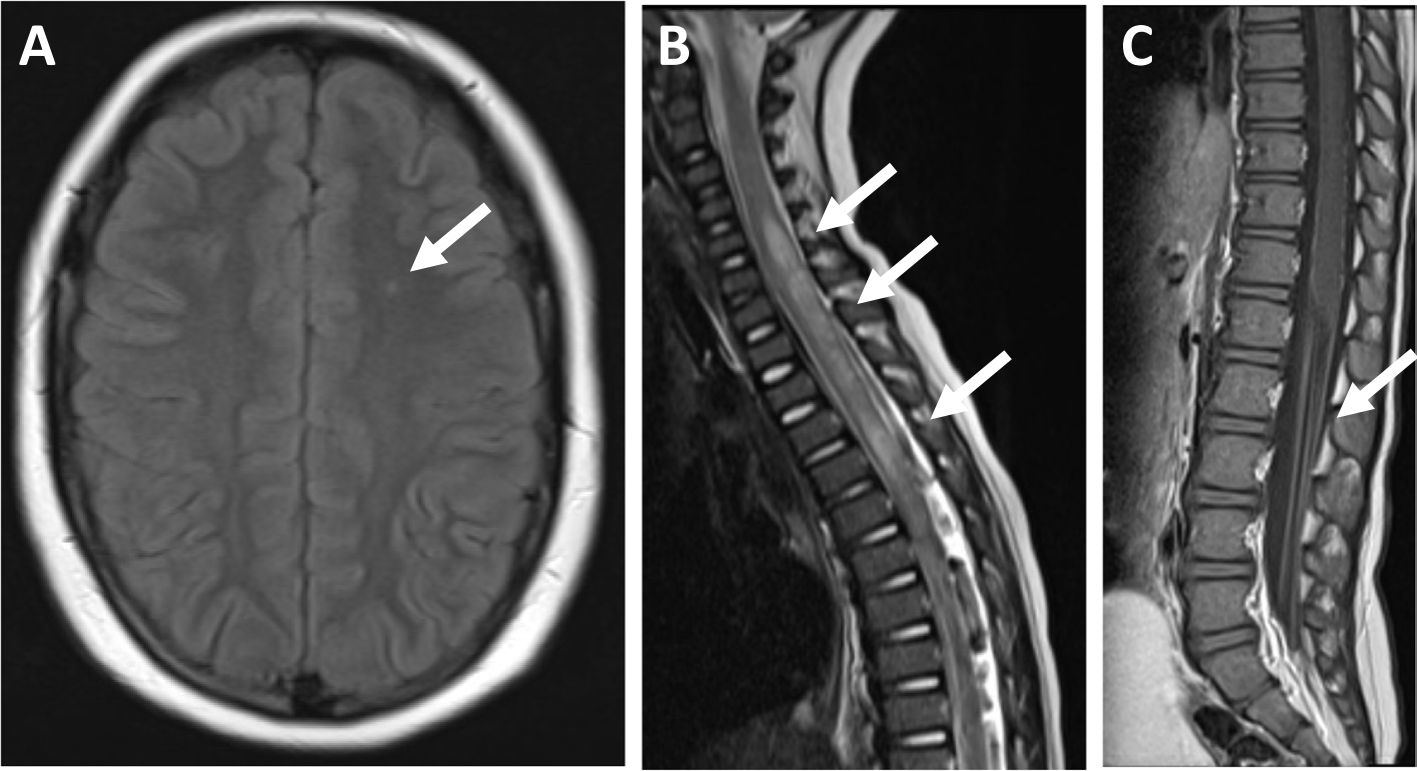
Immune-mediated neurological disorders in jSLE. A Demyelinating syndrome (DS) in a 15-year-old patient. Transversal MRI images showing increased spotty signals in frontal white matter in Fluid attenuated inversion recovery (FLAIR) sequences. B Myelopathy in a 10-year-old patient. Sagittal MRI with high-signal of the myelin in T2-weighted images. C Guillain-Barré syndrome in a 12-year-old patient. Contrast enhancement of the cauda equina; sagittal T1-weighed images
